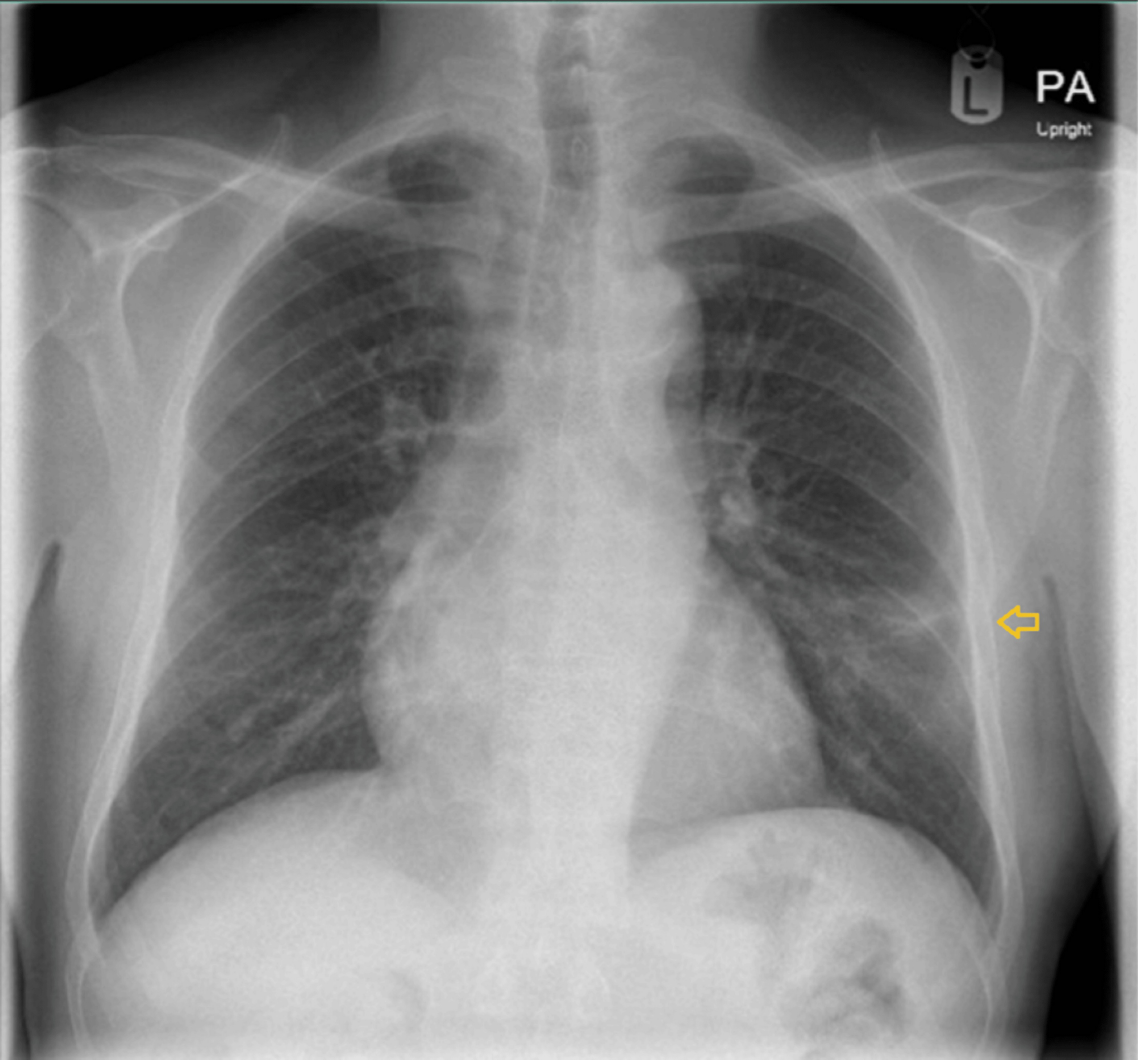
Follow-up X-ray after one month of treatment showed reduction in the density of left lower zone opacity (orange arrow).
